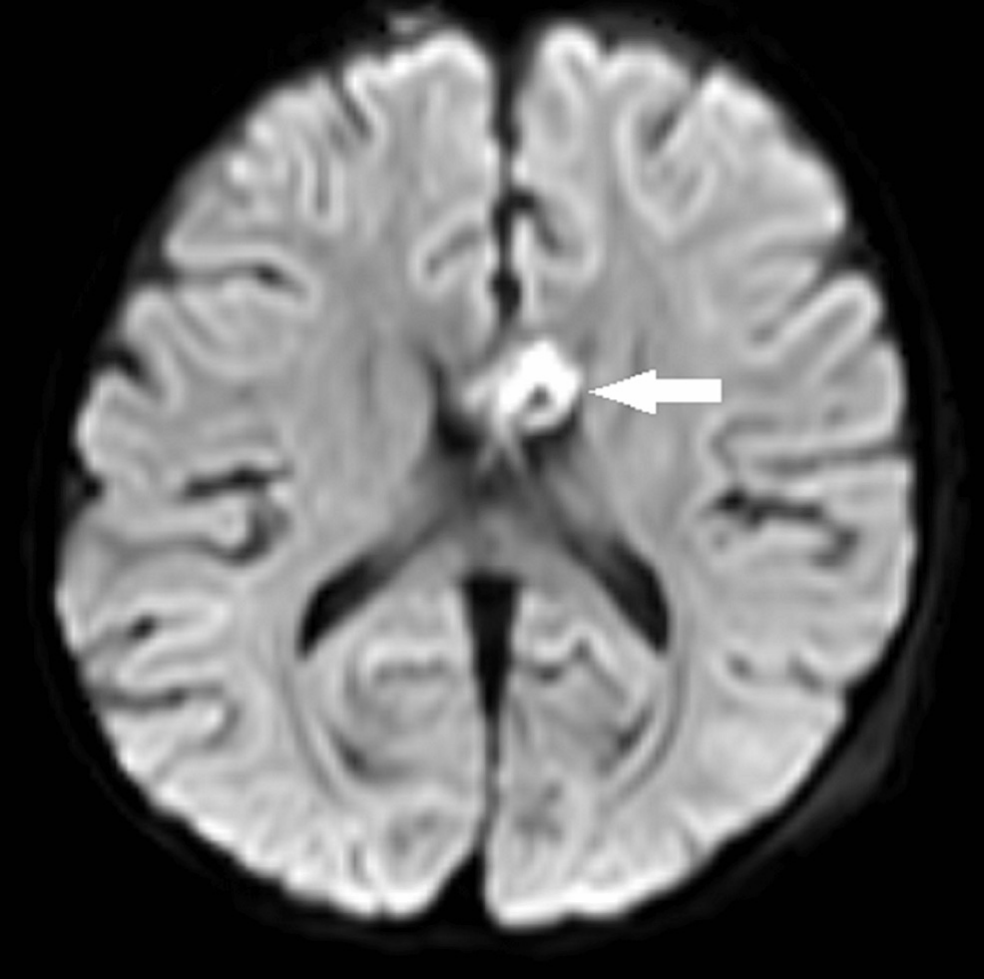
Axial MRI brain DWI image showing focus of hyperintense signal involving the genu of corpus callosum (white arrow) consistent with infarct DWI: diffusion-weighted imaging
